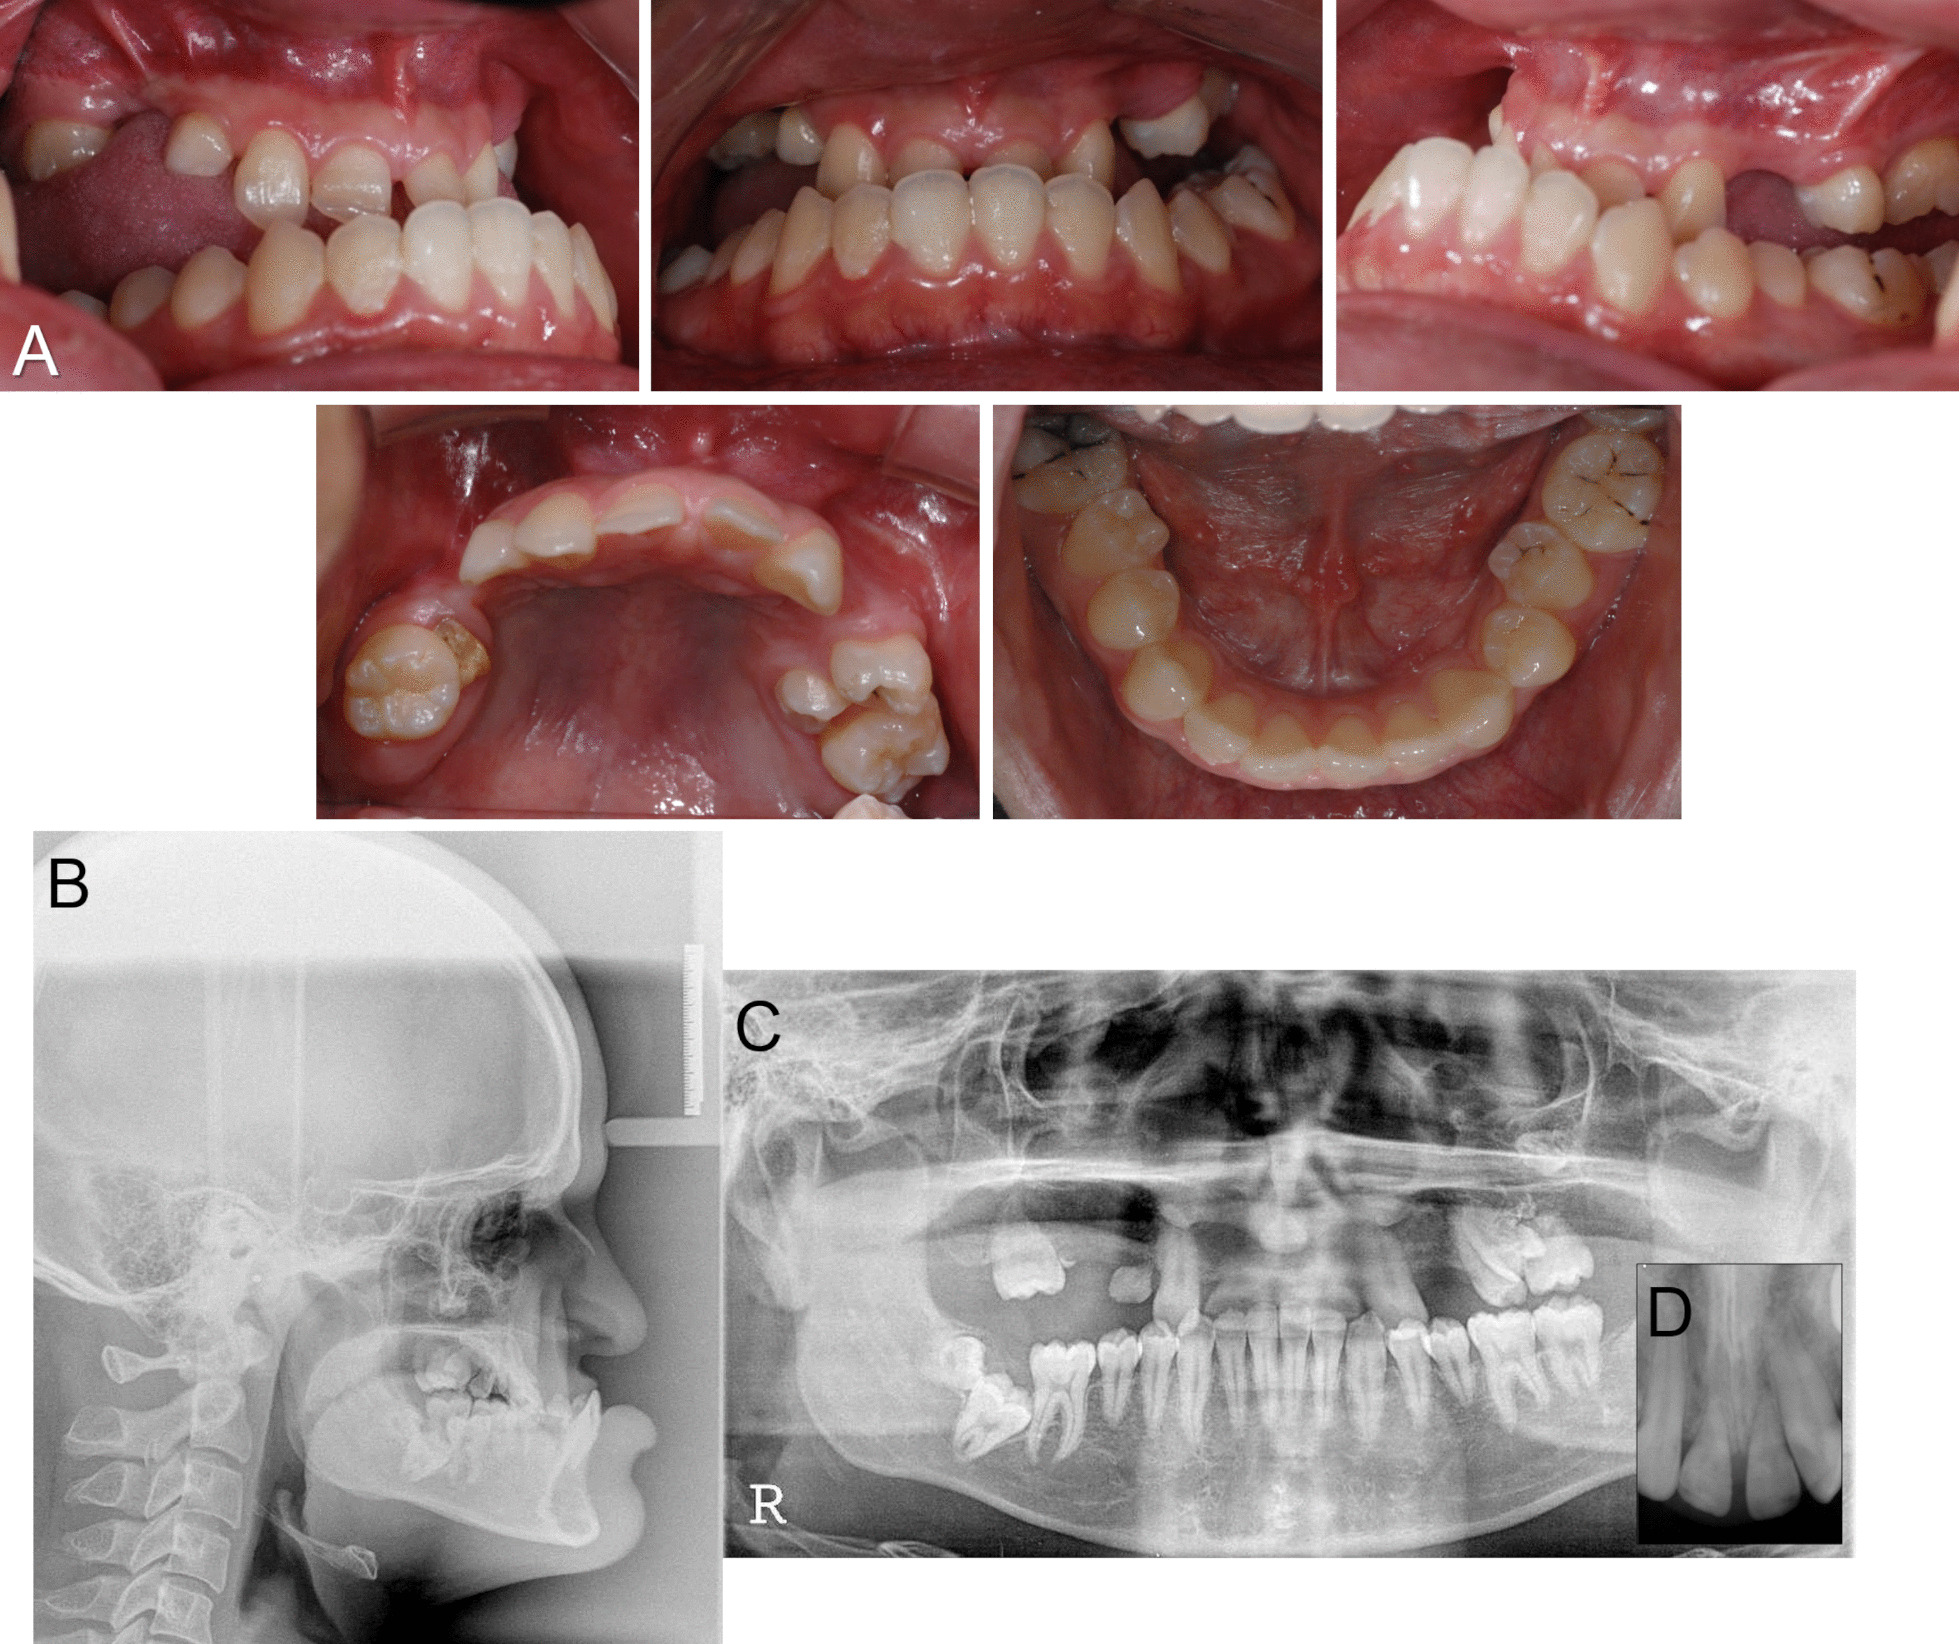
Pretreatment records of the patient. A. Intraoral photograph; B. Lateral cephalogram; C. Panoramic radiograph; D. Periapical radiograph
